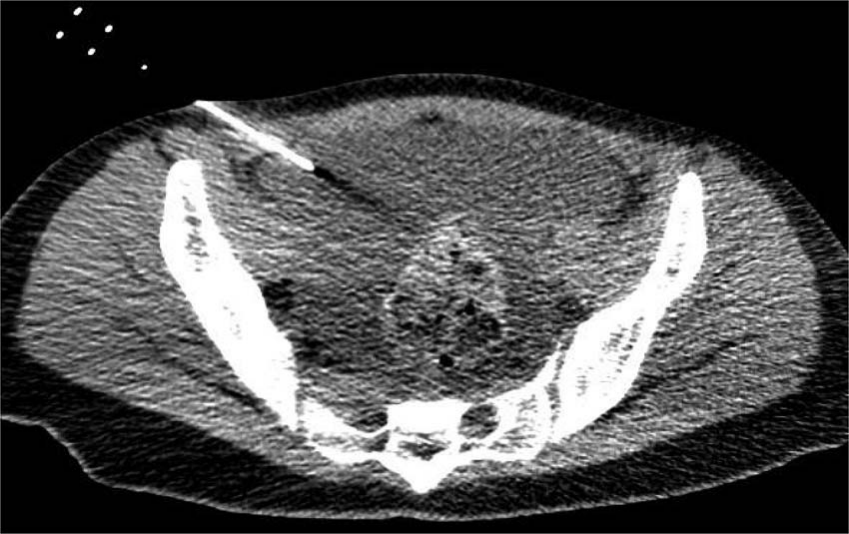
CT of the pelvic region on the fourth day. An increase in ascitic fluid was observed. CT-guided abdominal drainage was performed, and a 10 Fr drainage tube was placed.
